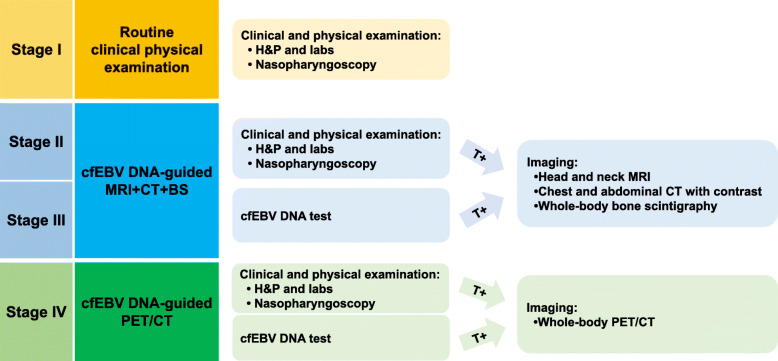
The cost-effective posttreatment surveillance strategy in each stage of nasopharyngeal carcinoma. The cfEBV DNA-guided MRI + CT + BS and cfEBV DNA-guided PET/CT refer to routine clinical and physical examinations and cfEBV DNA tests, followed by further imaging studies when the results are positive. Abbreviations: BS, bone scintigraphy; cfEBV, cell-free Epstein-Barr virus; CT, computed tomography; H&P, history and physical examination; MRI, magnetic resonance imaging; PET/CT, positron emission tomography/computed tomography; T, test
